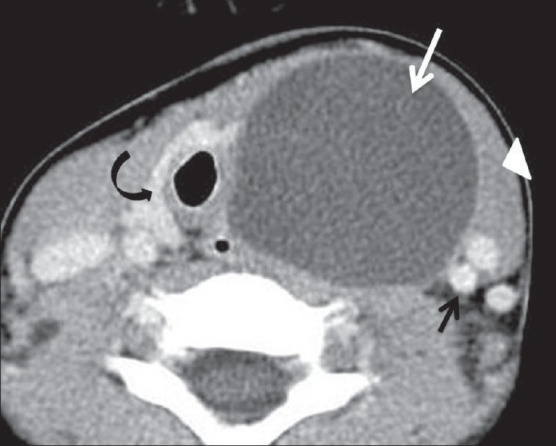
Axial CT scan of the neck shows the relationship of the lesion (arrow) with the sternocleidomastoid muscle (arrowhead), carotid vessels (short arrow), and the thyroid gland (curved arrow)
